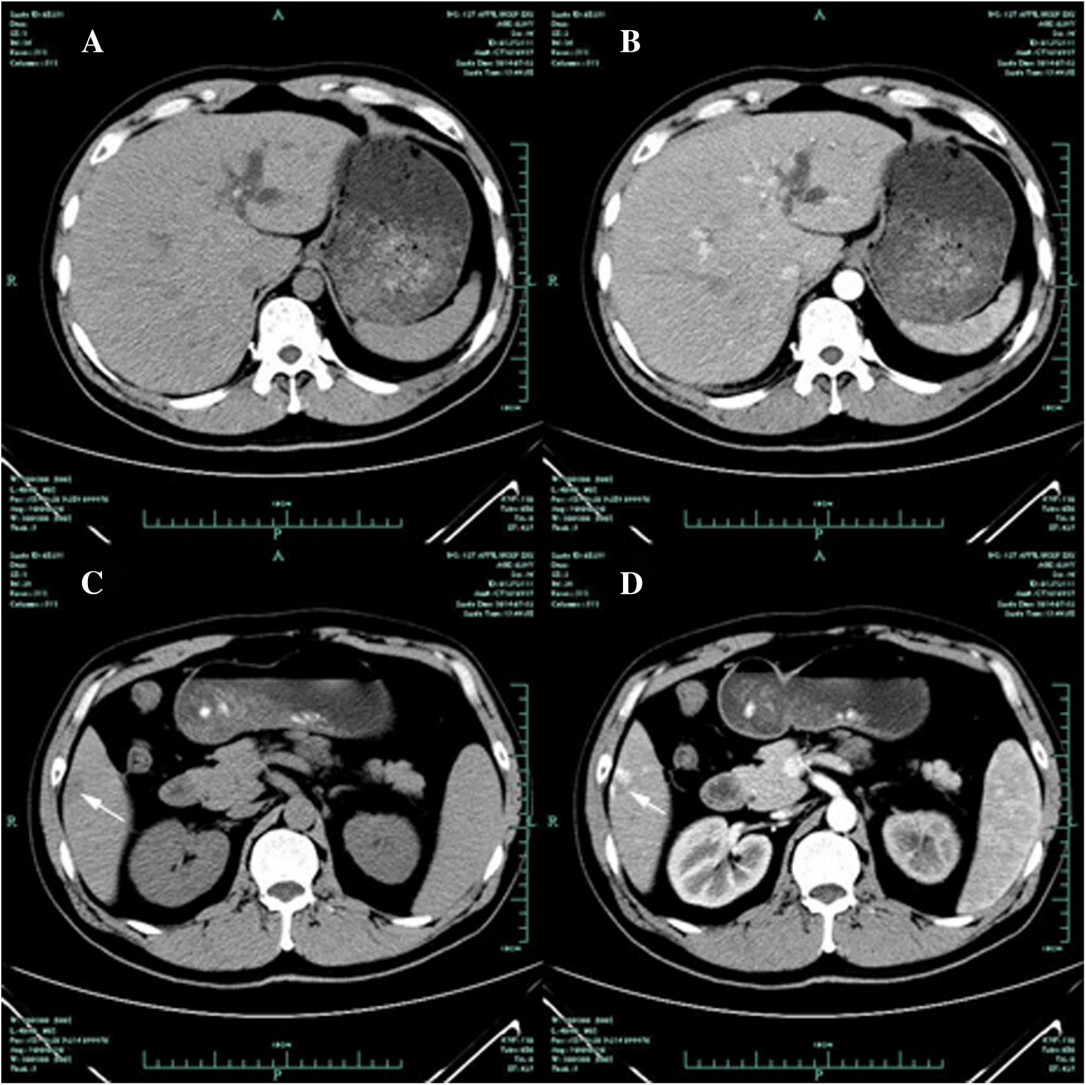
Abdominal computed tomography scan before surgery. a–b: Abdominal computed tomography scan (plain and arterial phase) revealed multiple cholesterol stones in intrahepatic bile ducts of the left hepatic lobe concomitant with intrahepatic cholangiectases; c–d: A low-density mass (arrow) with an estimated magnitude of 2.0 × 1.8 cm and a vague margin was also observed
